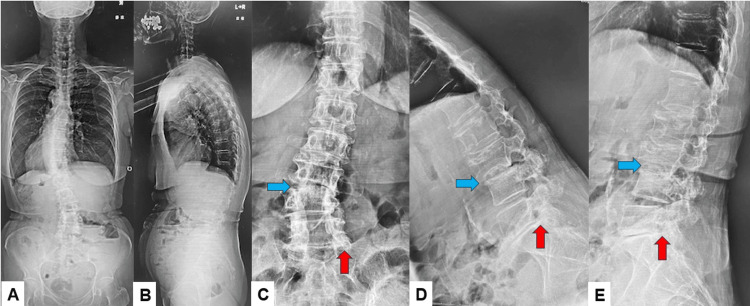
Preoperative radiographs. Preoperative radiographs showing L3-4 collapse with associated endplate changes. A: Anteroposterior whole-spine radiograph; B: lateral whole-spine radiograph; C: anteroposterior lumbar radiograph; D: lateral flexion lumbar radiograph; E: lateral extension lumbar radiograph. Red arrows indicate left foraminal narrowing. Blue arrows indicate L3/4 angulation and instability.
